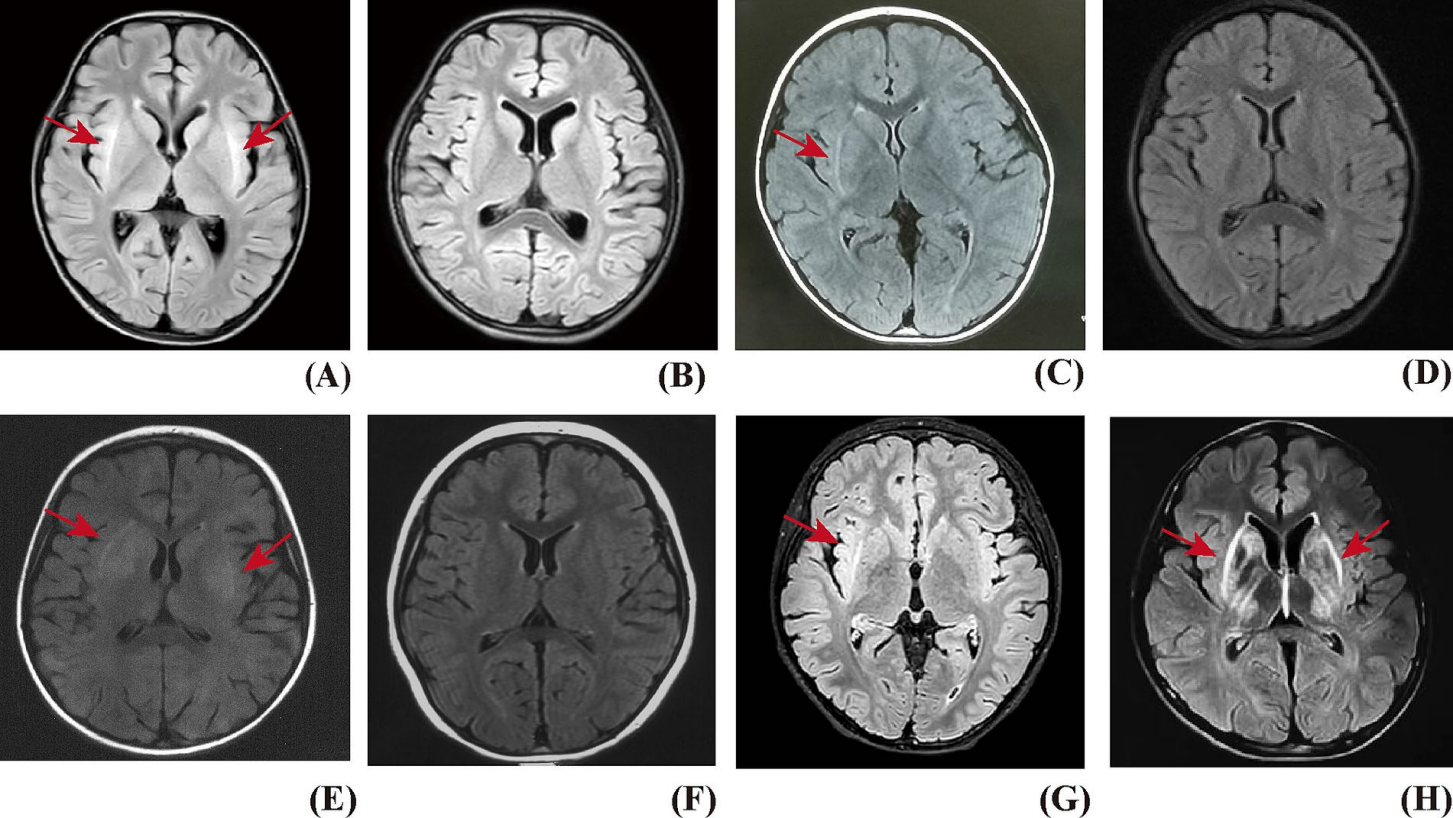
The claustrum sign on T2 FLAIRE of brain MRI. (A) Patient with FIRES, bilateral claustrum hyperintensity presented on MRI. (B) The claustrum sign disappeared on the follow-up MRI. (C) Patient with AbNAE, right claustrum hyperintensity presented on MRI, with co-occurring lesions in the head of the caudate nucleus and the putamen. (D) The claustrum sign disappeared on the follow-up MRI. (E) Patient with MOGAD, bilateral claustrum hyperintensity presented on MRI, with co-occurring lesions in the bilateral head of the caudate nucleus, putamen, and thalamus. (F) The claustrum sign disappeared on the follow-up MRI. (G) Patient with AbNAE, showed right claustrum hyperintensity presented on MRI, which persisted on the follow-up MRI on Day 640. (H) Patient with WD, bilateral claustrum hyperintensity presented on MRI, with co-occurring lesions in the head of the caudate nucleus and the putamen, which persisted on the follow-up MRI on Day 162.
